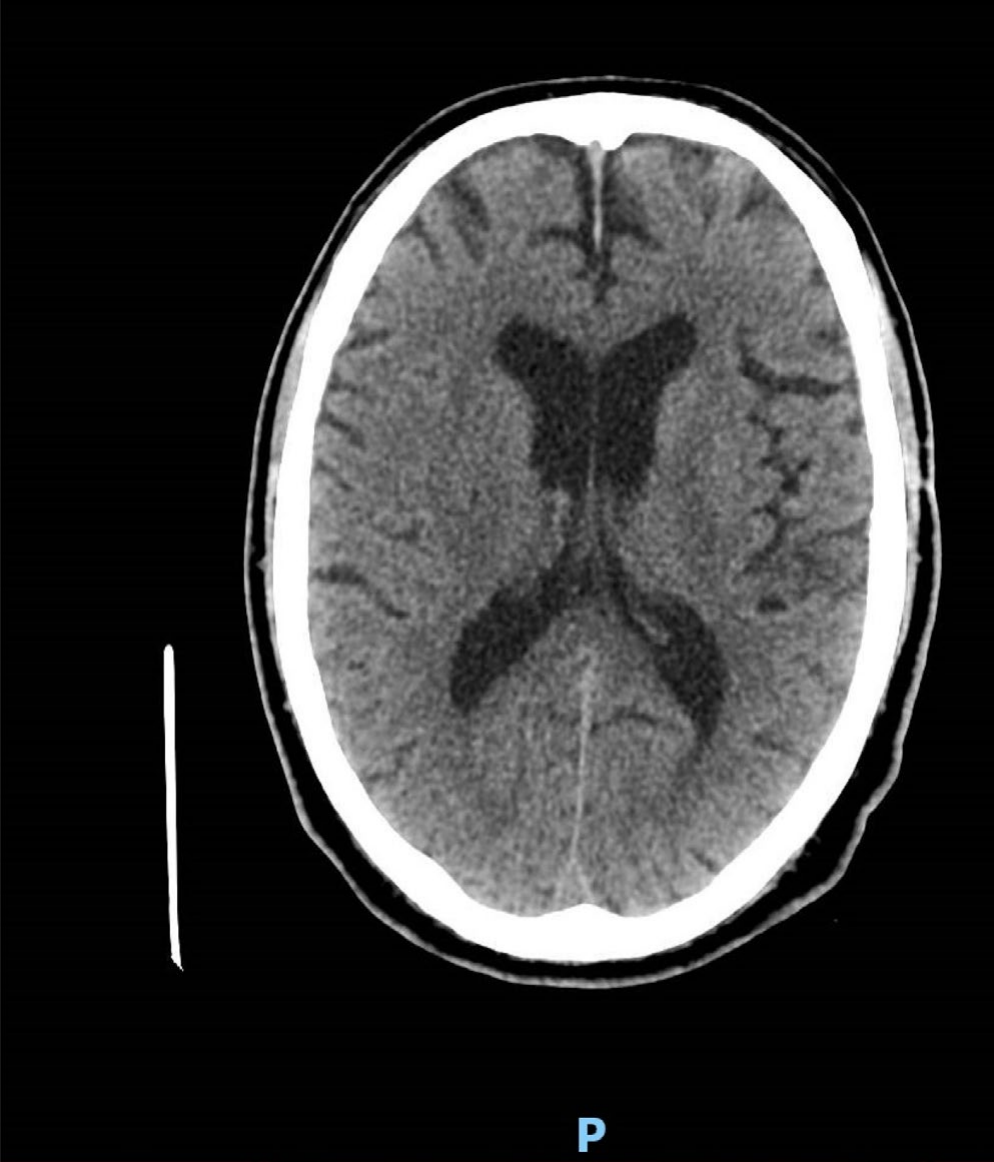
Head computed tomography showing normal scan
